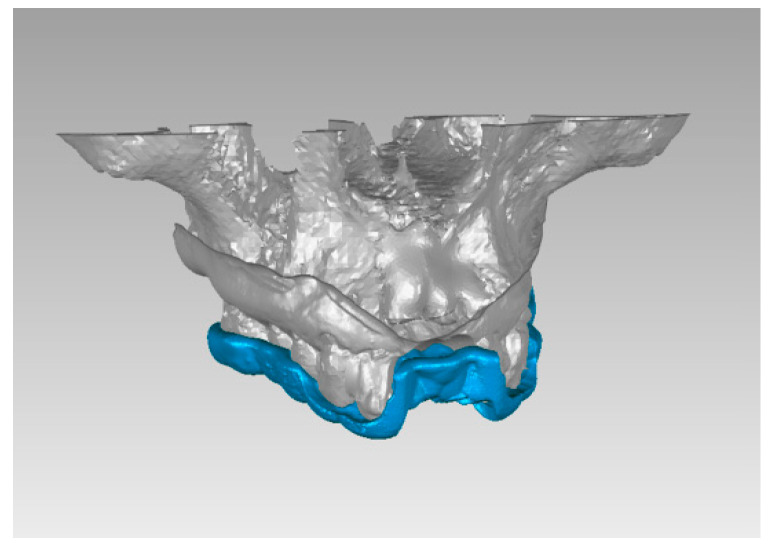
Matching of pre-operative CT, plaster model, and pilot drill template.
